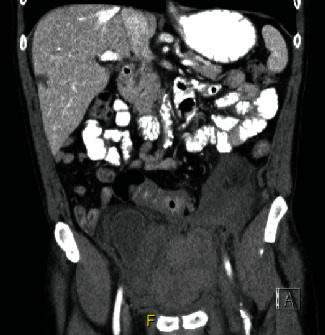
Initial CT abdomen and pelvis in ED (coronal): 16 hours postop with large pelvic hematoma.
